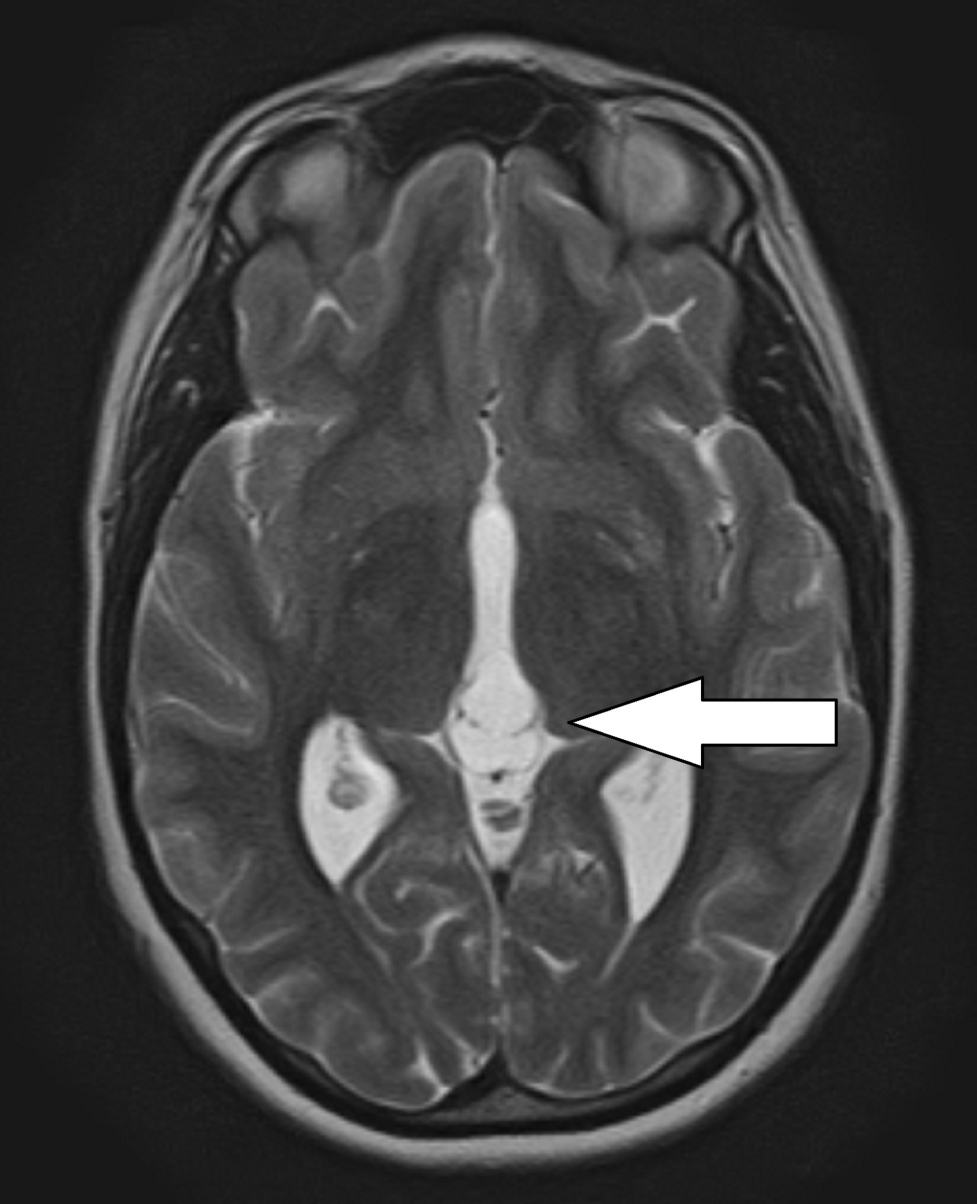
T2-weighted axial magnetic resonance imaging (MRI) demonstrating septated pineal cyst.
